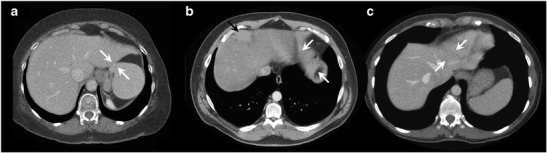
Imaging pitfalls which may limit liver segmentation on CT. a Axial enhanced CT image of a 62-year-old woman shows indistinct liver-spleen boundaries (*arrows*). b Axial enhanced CT image of a 47-year-old man depicts segmentation challenges caused by ill-defined and non-continuous borders found near the liver dome (*arrows*). c Axial enhanced CT image of a 73-year-old man shows partial volume averaging between the left liver and the heart (*arrows*) [13]
